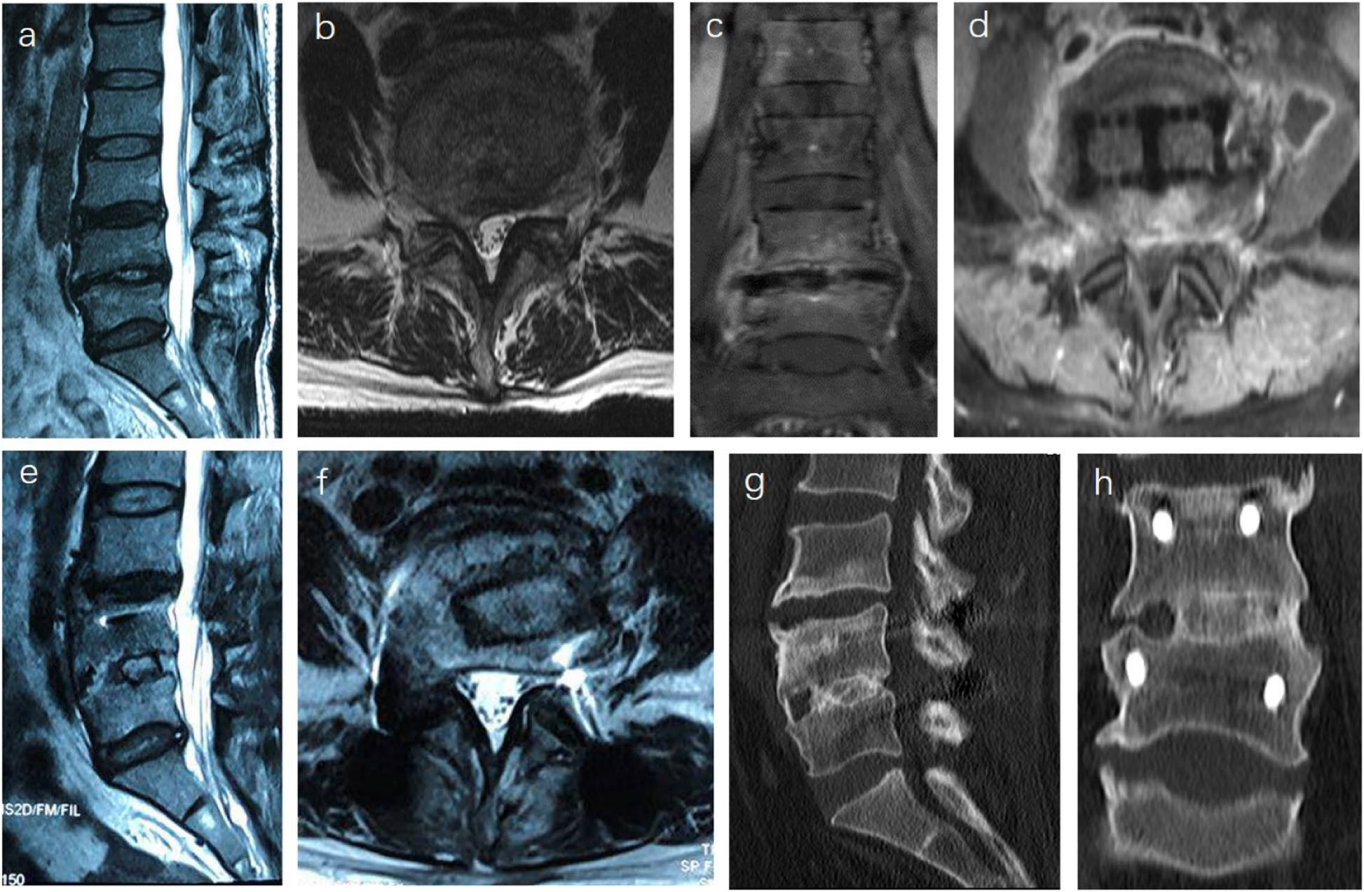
A case of intervertebral space infection. a, b Preoperative sagittal and axial MRI. c, d Coronal and axial MRI showed infection in L45 and intervertebral space collapse. e, f Sagittal and axial MRI after debridement and posterior internal fixation. g, h Sagittal and axial CT at one year after operation showed that bony fusion had been achieved
